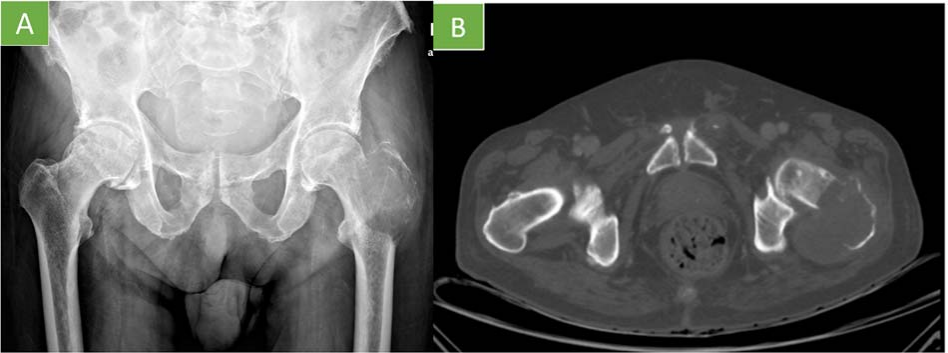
(A) X-ray pelvis AP view: geographic lytic insufflating expansive lesion centered in the intertrochanteric region of the left femur that destroys the cortex with poorly defined borders in certain locations. Soft tissue mass associated. The tumor matrix shows thin septum reaching 9 cm in longitudinal axis. (B) Axial CT: lytic lesion with cortical destruction at trochanteric level.
